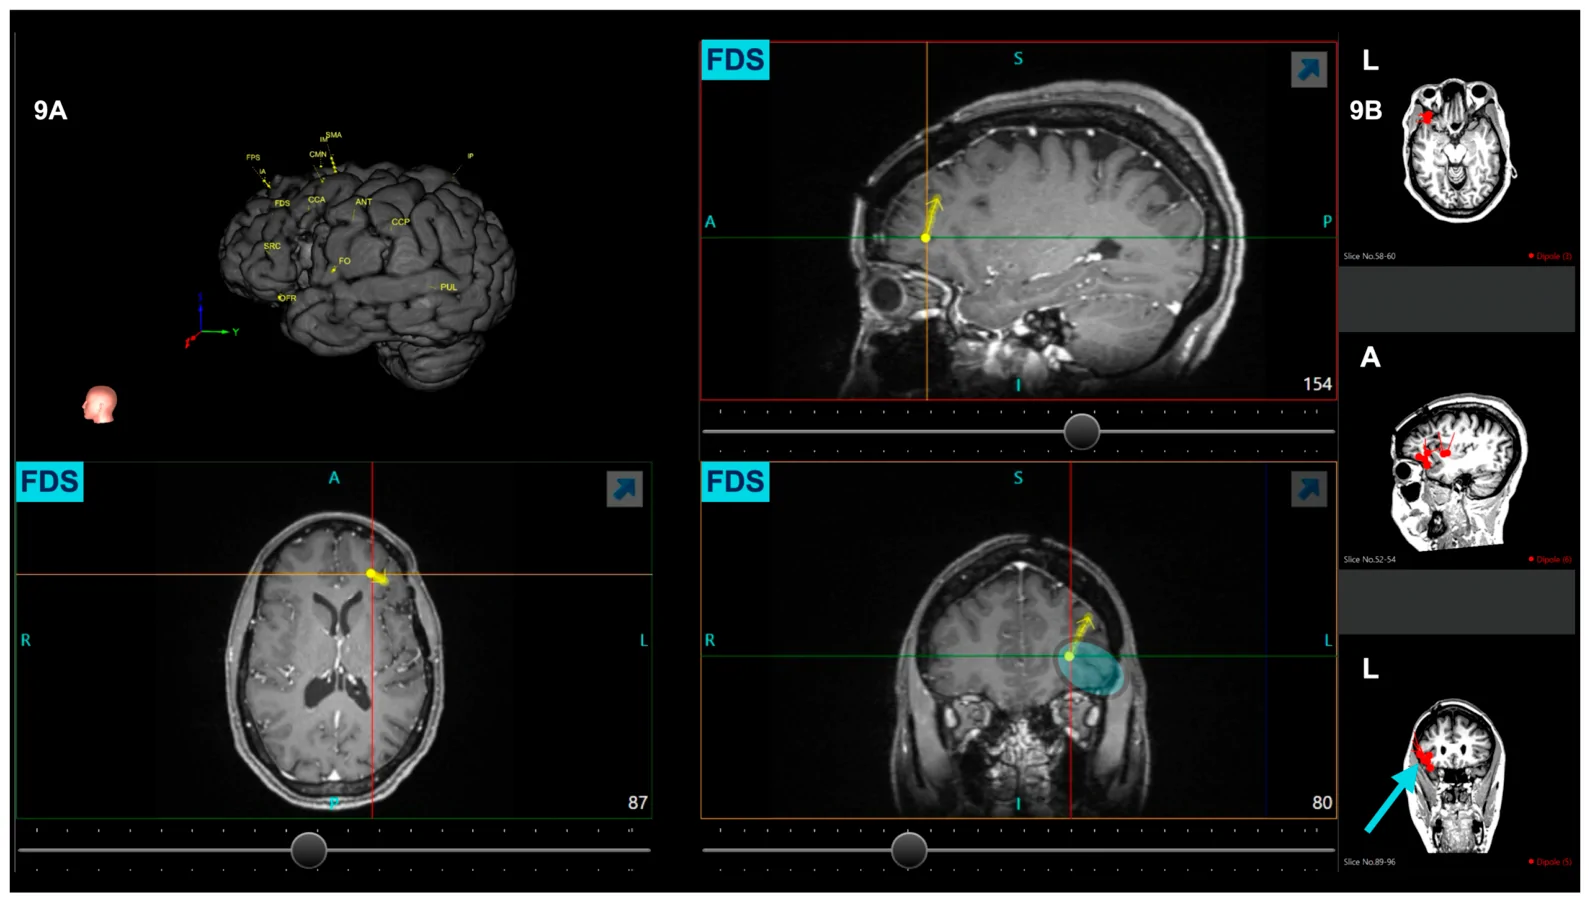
(A,B) SEEG and MEG findings. (A) is the three-dimensional reconstruction of the SEEG implantation (upper left panel) and co-registration of the SEEG depth electrode within the patient’s brain MRI (yellow arrow; FDS—frontal dysplastic sulcus). The light blue oval outlines the irregular gyrus and sulcus within the left orbitofrontal region that several MEG ECDs mapped to and from which spontaneous seizures emanated on SEEG. (B) is the cluster of MEG ECD mappings to the abnormal gyrus and sulcus in the left orbitofrontal region (light blue arrow). The findings in this study reinforce the published literature insofar as MEG ECD can accurately identify epileptogenic brain regions [21,22,54,55,57,58,59,62,63,66,67], identify previously underappreciated cortical malformations [60,61,63], accurately localize a seizure onset zone [64], and augment the semiology and SEEG implantation strategy [22,55,57,68].
